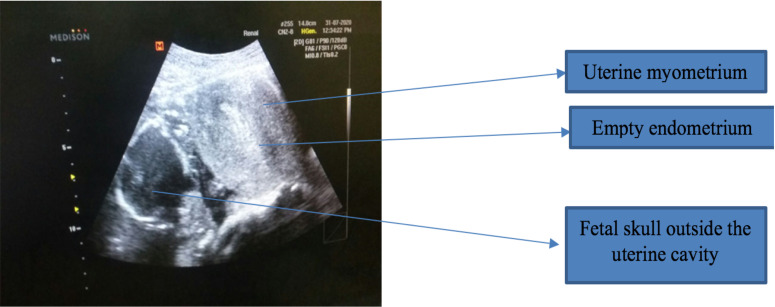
Ultrasound of the abdomen showing empty uterus with fetal parts in the abdominal cavity.
